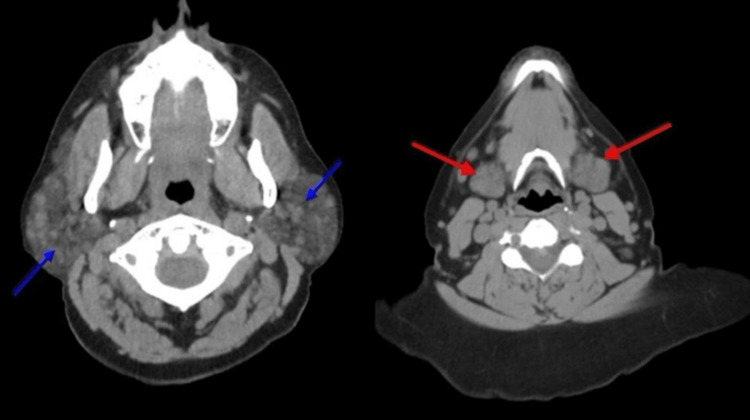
CT neck without contrast. Blue arrows showing enlarged parotid glands and innumerable lymph nodes, red arrows showing enlarged submandibular glands CT: Computed tomography
